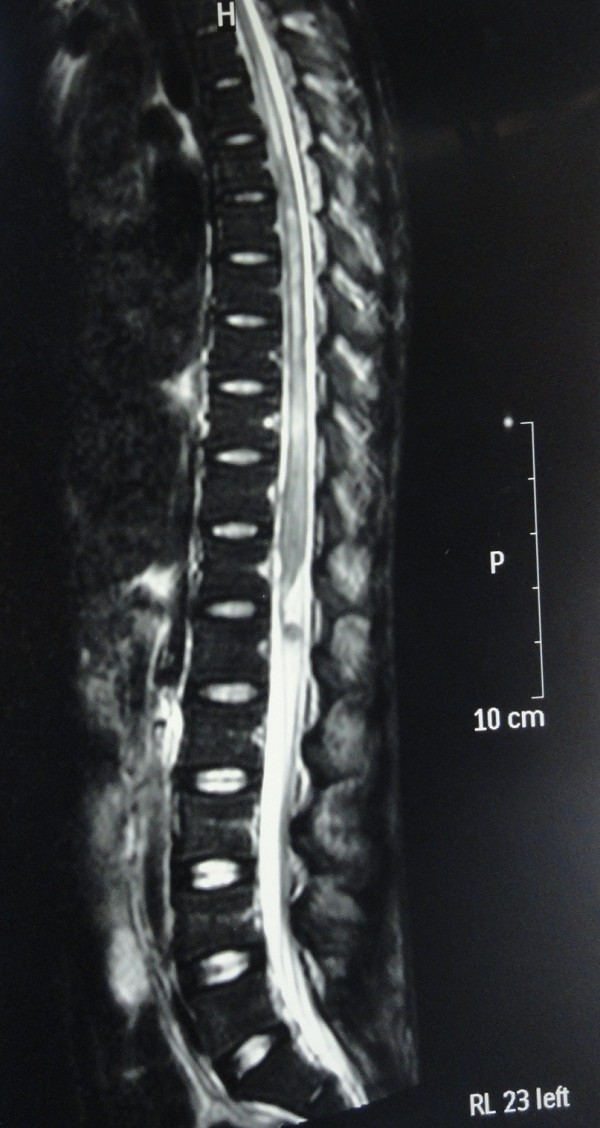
MRI thoracolumbar spine showing extensive myelitis. MRI thoracolumbar spine shows extensive involvement of the spinal cord from T4 downwards with foci of T2W hyperintensity and meningeal enhancement.
